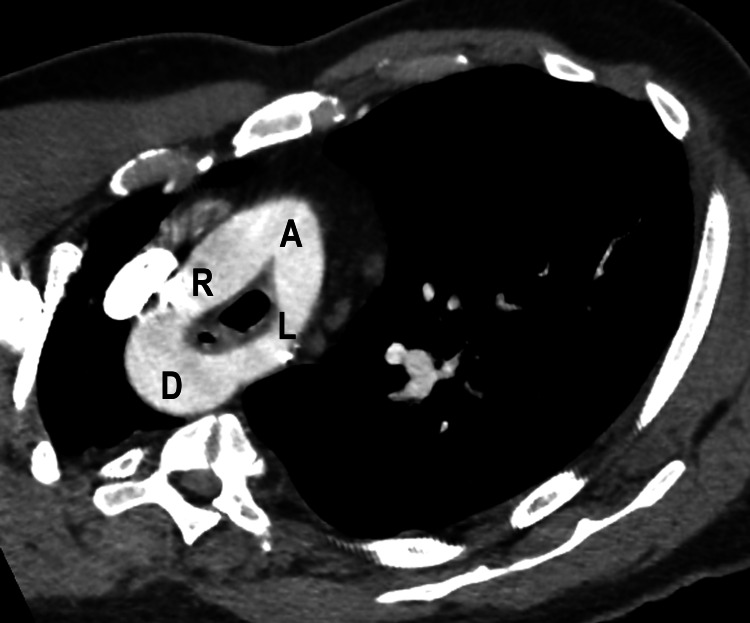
Oblique axial reformatted computed tomography image depicts a vascular ring with the ascending aorta (A) dividing into the right (R) and left (L) arch components which merge to form the descending thoracic aorta (D). Note how the ring surrounds the trachea and constricts the esophagus.
